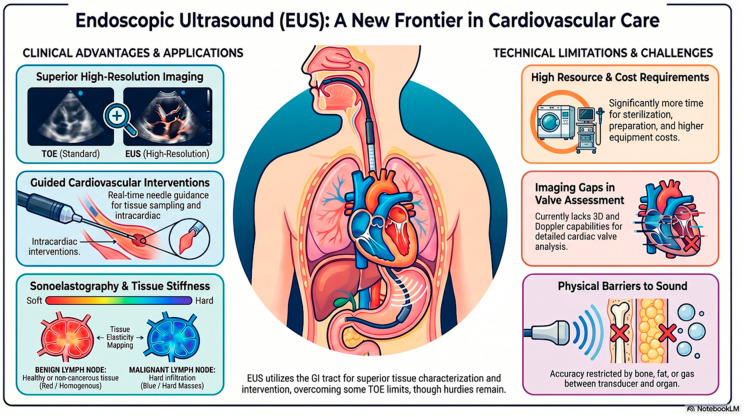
Summary figure of endoscopic ultrasound potential indications, advantages and disadvantages in cardiovascular medicine. Image created using NotebookLM, Google, April 2026.
